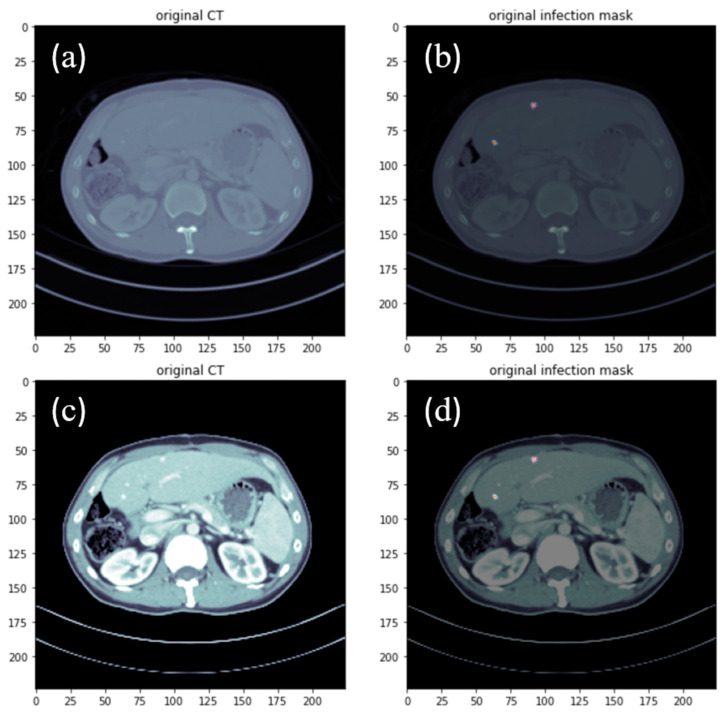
Before adjustment of HU values from the (a) original hospital CT image data and (b) annotated lesion image data (white colored is the location of the lesion); after adjustment of HU values from the (c) original hospital CT image data and (d) annotated lesion image data (white colored is the location of the lesion).
